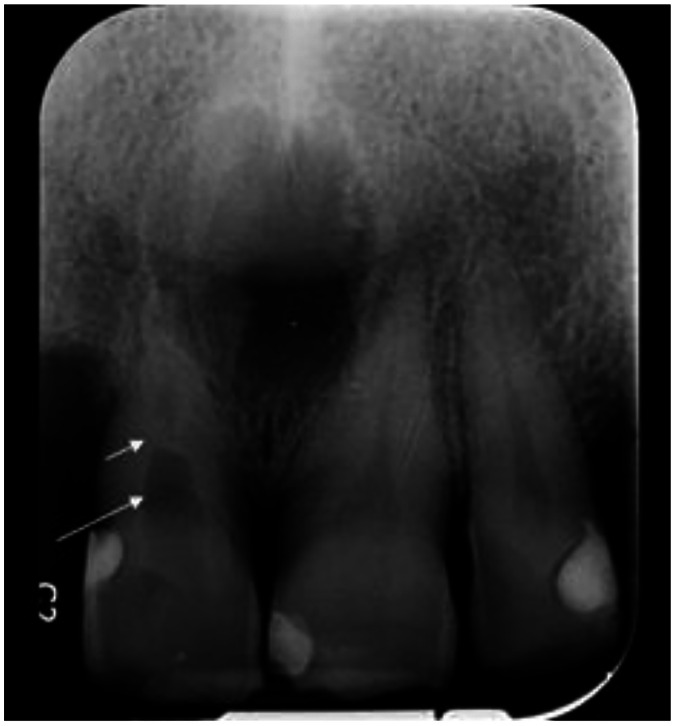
Initial Periapical radiography. Dentin bridge (short arrow), internal root resorption (long arrow).
